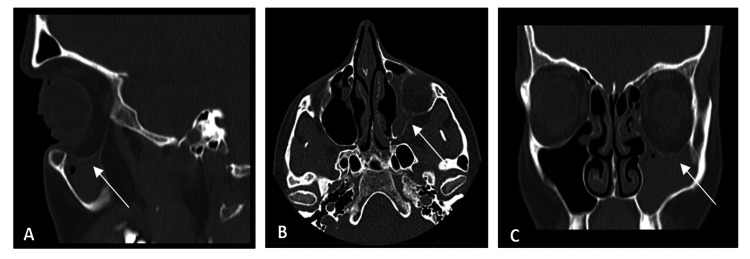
Preoperative CT scan showing the completely opacified left maxillary sinus, descent of the left orbital floor, and a decrease in the thickness of the inferior rectus muscle. A) Sagittal CT section showing the descent of the orbital floor and occupation of the left maxillary sinus (arrow). B) Axial section of the CT showing atelectasis of the left sinus volume (arrow) compared to the contralateral. C) Coronal section of the CT showing on the left side the smaller size of the inferior rectus muscle, the bone thinning of the orbital floor, and the occupation of the maxillary sinus (arrow). CT: Computed tomography
